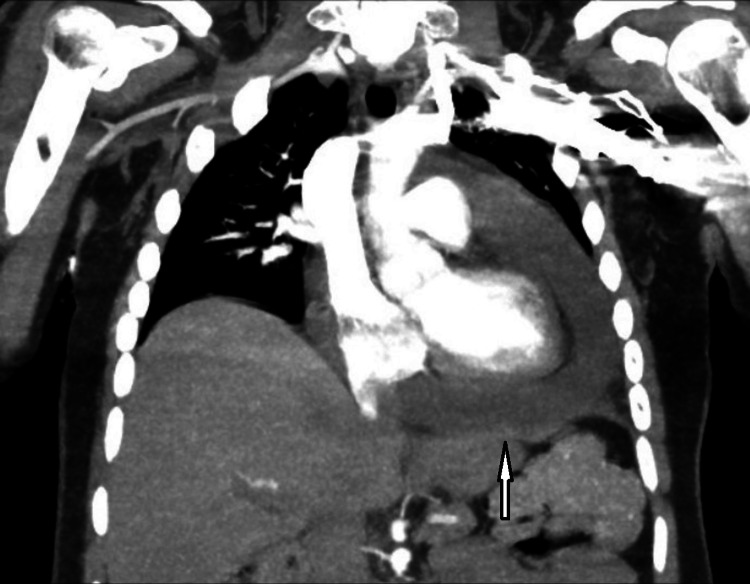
CT angiography chest showing a large pericardial effusion, which is concerning for a cardiac tamponade. CT: Computed tomography
